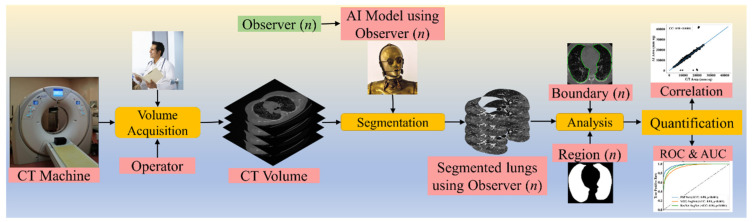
COVLIAS 1.0: Inter-variability analysis of CT-based lung segmentation and quantification system for COVID-19 patients. ROC: Receiver operating characteristic; AUC: Area-under-the-curve.
